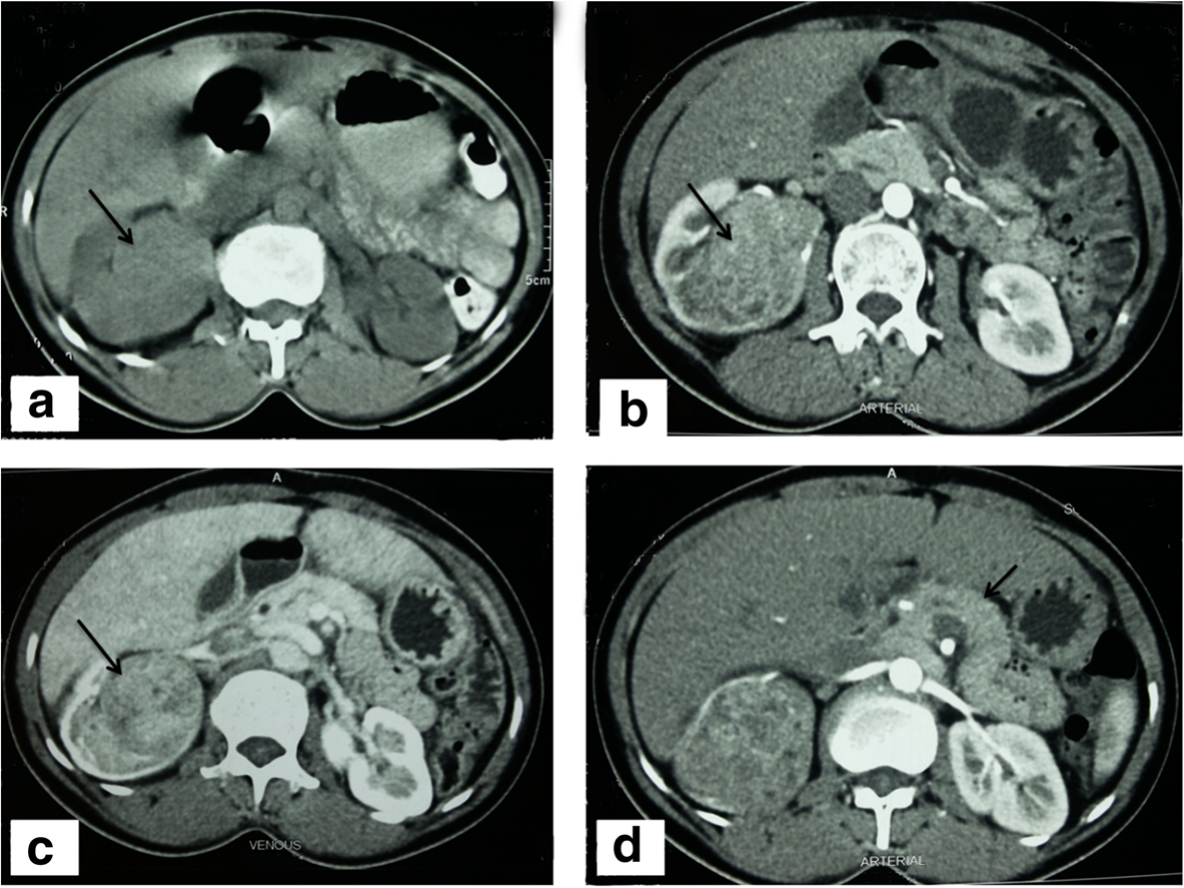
CT scan. Unenhanced CT axial image (1a) showing an isodense mass (arrow) to renal parenchyma which show enhancement in arterial phase image (1b) but lesser than the renal cortex. The enhancement continued till the venous phase (1c). Arterial phase image (1d) taken at the level of pancreas (arrow) did not show any arterial enhancing lesion.
